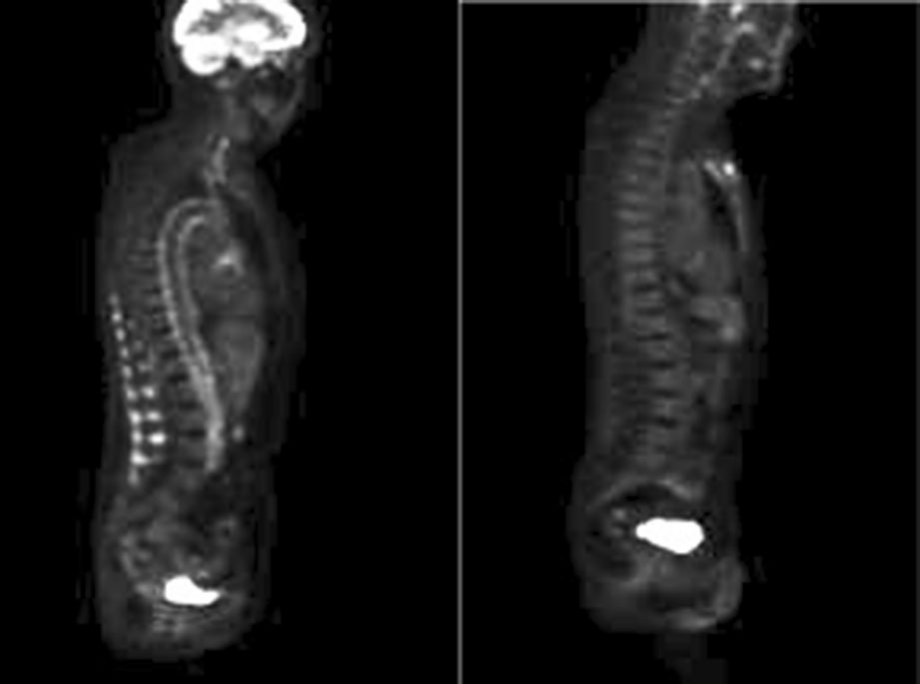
(Left) Sagittal FDG PET image of a patient with active aortitis. (Right) Sagittal FDG PET image of a control patient
